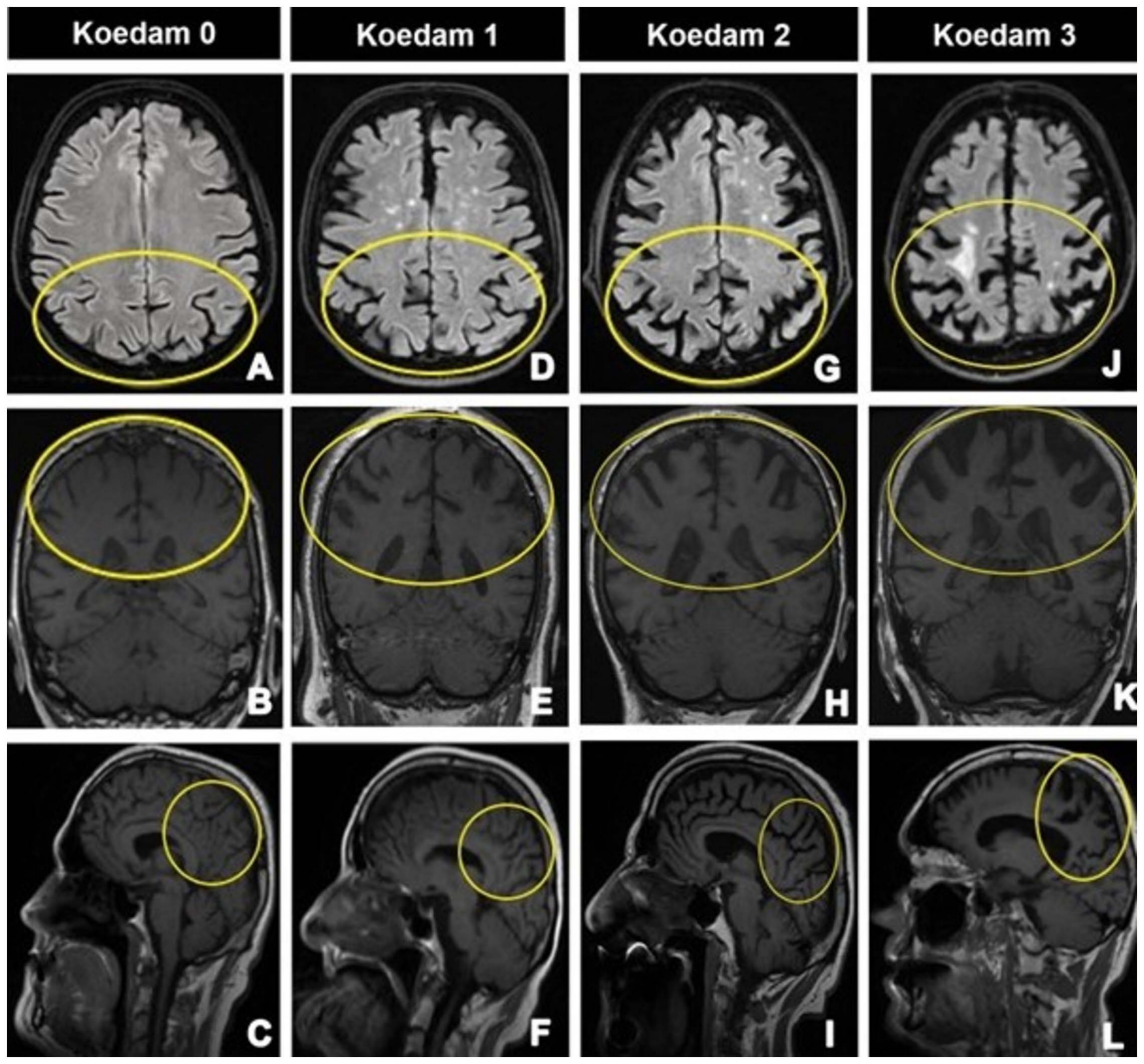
Axial FLAIR, coronal T1W, and sagittal T1W images show parietal atrophy scale. Images are of 4 different patients with Alzheimer’s disease, aged 70, 87, 86, and 83 years. First column: Koedam 0: (A)–(C) show the closed posterior cingulate, parieto-occipital, and the parietal lobe sulci, and the normal precuneus (yellow circles). Second column: Koedam 1: (D)–(F) show mild posterior cingulate, parieto-occipital, and the parietal lobe sulcal widening, and the mild atrophy of precuneus (yellow circles). Third column: Koedam 2: (G)–(I) show substantial posterior cingulate, parieto-occipital, and the parietal lobe sulcal widening, and the substantial atrophy of precuneus (yellow circles). Fourth column: Koedam 3: (J)–(L) show extremal posterior cingulate, parieto-occipital, and the parietal lobe sulcal widening, and the mild atrophy of precuneus, knife-blade precuneus atrophy (yellow circles). FLAIR: Three-dimensional T2-weighted fluid-attenuated inversion-recovery imaging.
